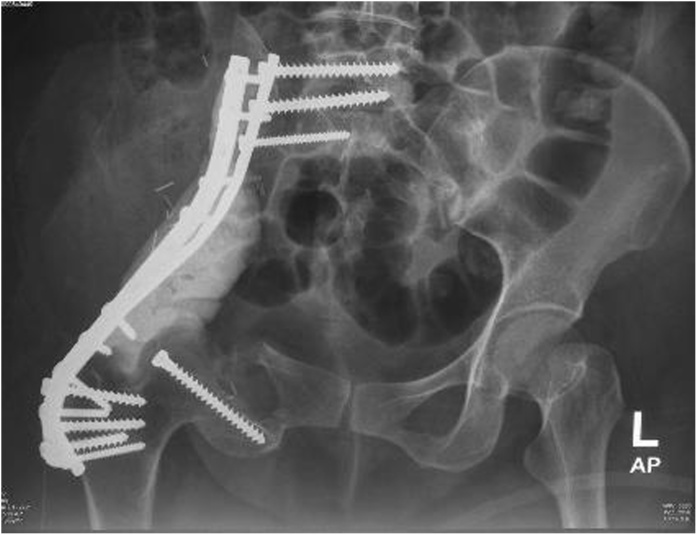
Postoperative X-Ray Examination Showing Plate, Screw, and Bone Cement after Reconstruction Procedure.
